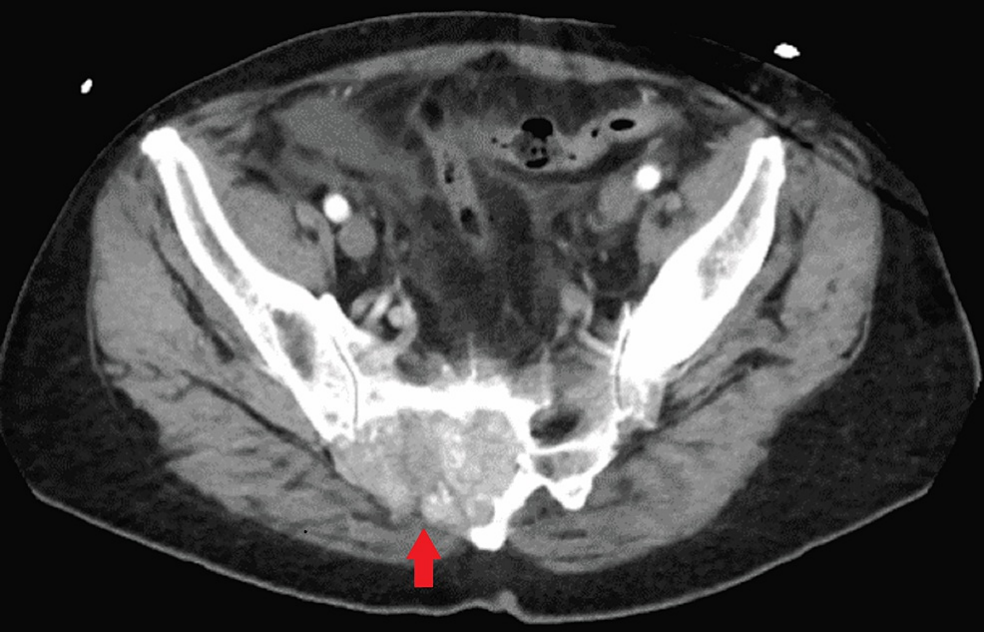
Pelvic CT (transverse view) revealing right sacral deep-seated mass
